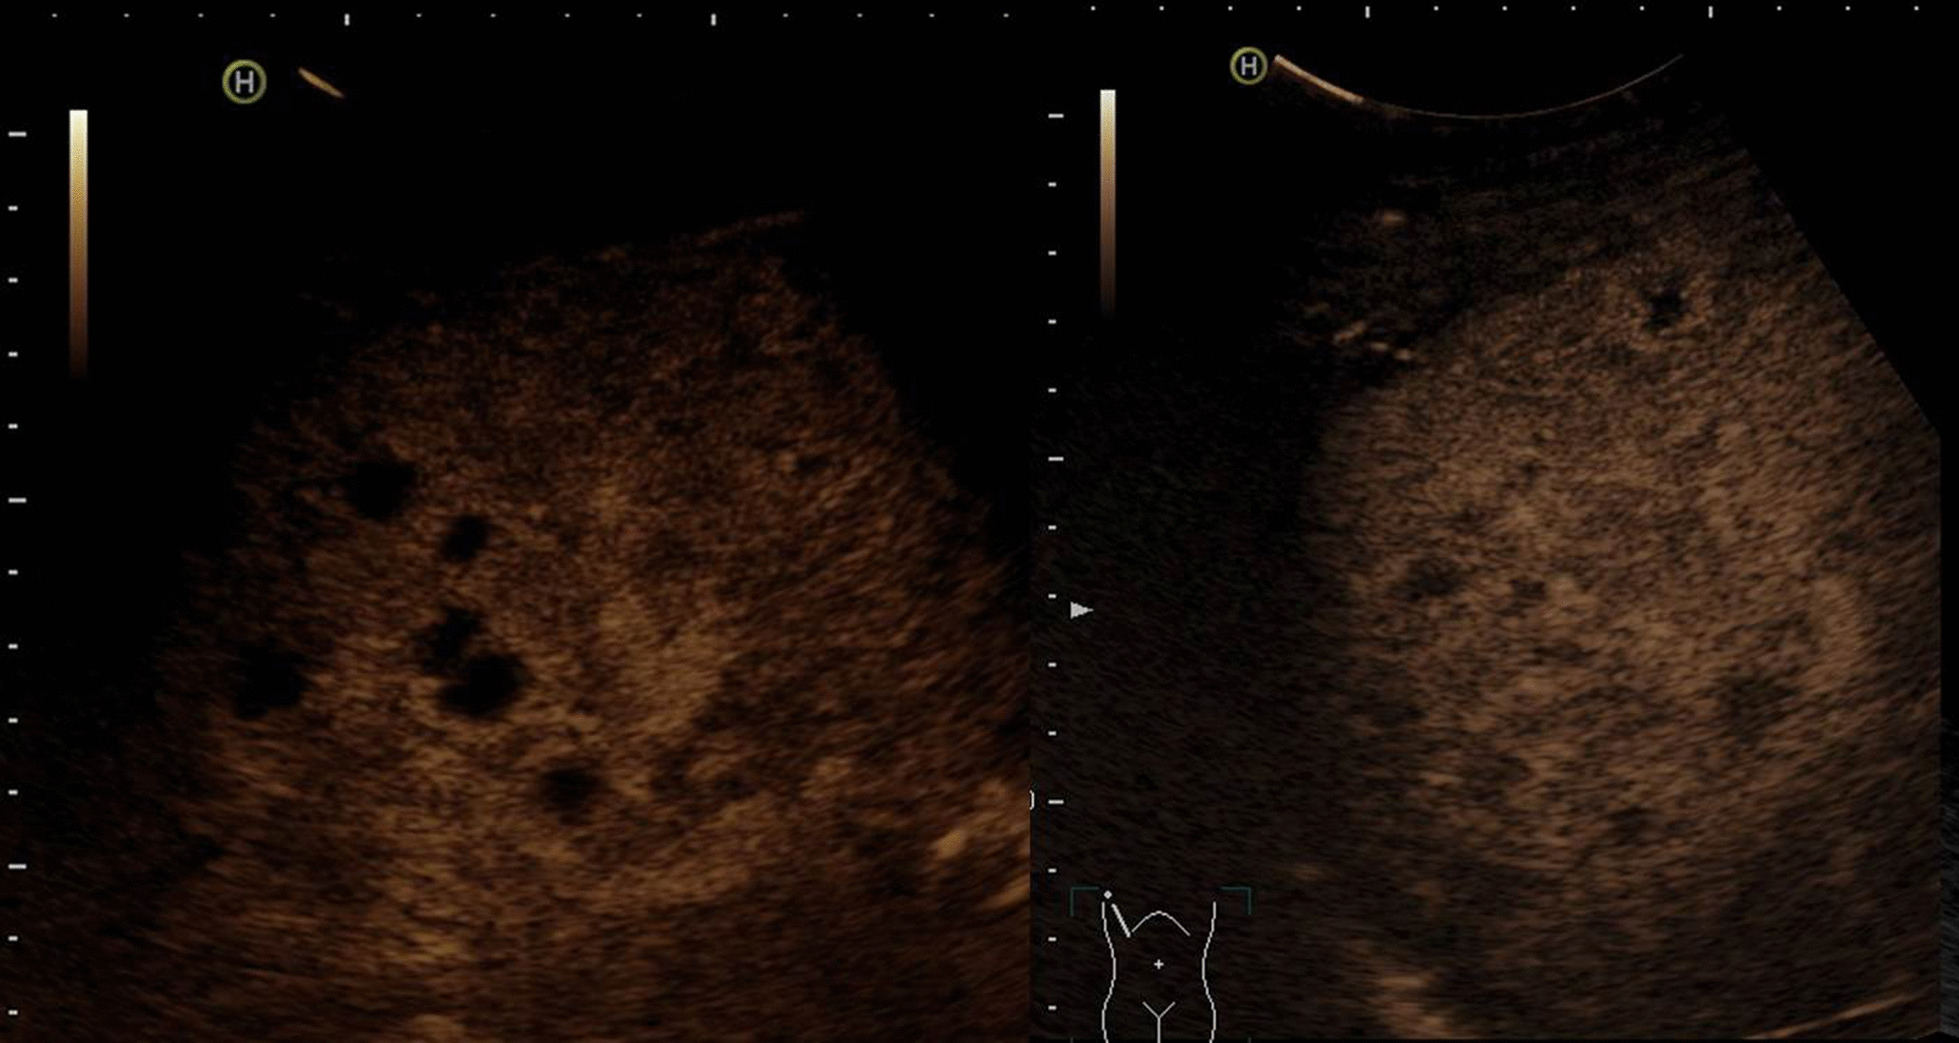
Contrast-enhanced ultrasound with suggested rim-like hyperechogenic contrast uptake due to hyperemia around the abscesses (stage IV). Due to missing arterial perfusion, there is no contrast uptake in the necrotic hypoechogenic lesions
